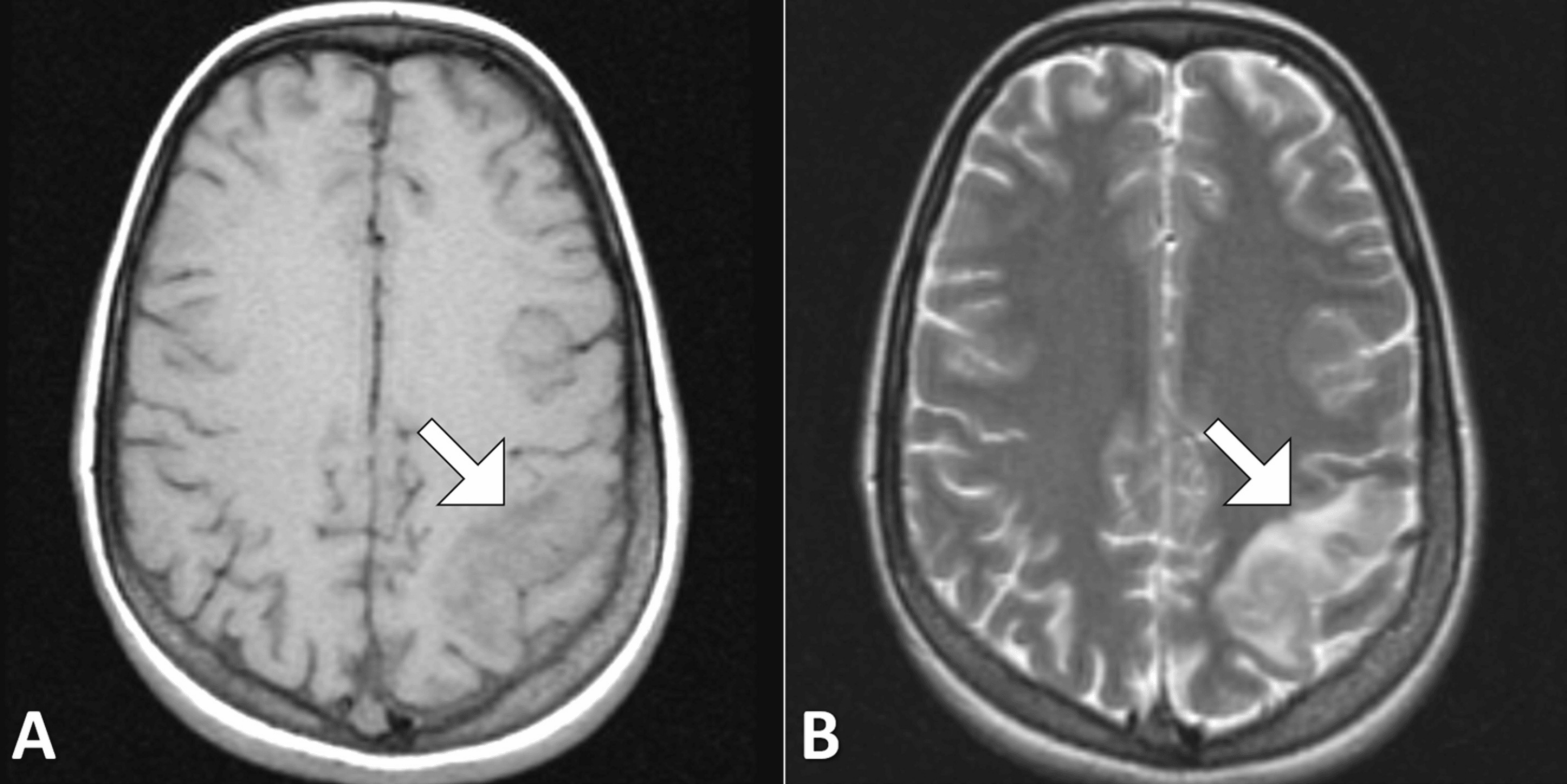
Axial T1- and T2-weighted MRI of the left parietal lobe. Axial T1-weighted MRI (A) shows a hypointense lesion (white arrow) in the left parietal cortex. Corresponding axial T2-weighted MRI (B) demonstrates hyperintensity at the same location (white arrow), mimicking a neoplastic lesion. T1 hypointensity and T2 hyperintensity without mass effect are suggestive but non-specific.
